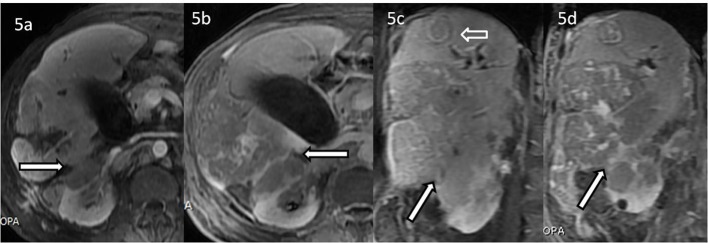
Post contrast MRI sequences describe the loss of fat planes between the tumour and right kidney. (a) Axial late arterial phase post contrast MRI T1-weighted fat suppressed sequence showing the loss of fat planes between right renal upper cortex and the liver mass in the right lobe of liver showing mild enhancement but relatively hypointense to the liver (bold white arrow with black outline). (b) Axial portal venous phase post contrast MRI T1-weighted fat suppressed sequence showing the loss of fat planes between right renal upper cortex and the liver mass in the right lobe of liver (bold white arrow with black outline). (c) Coronal equilibrium phase post contrast MRI T1-weighted fat suppressed sequence showing the loss of fat planes between right renal upper cortex and the liver mass in the right lobe of liver appearing enhanced, however hypointense compared to the liver parenchyma (bold white arrow with black outline). Note is made of another target like lesion in the liver (white arrow). (d) Coronal delayed (1 h) phase post contrast MRI T1-weighted fat suppressed sequence showing the loss of fat planes between right renal upper cortex and the liver mass in the right lobe of liver showing areas of enhancement, however appearing hypointense compared to the liver parenchyma (bold white arrow with black outline).
